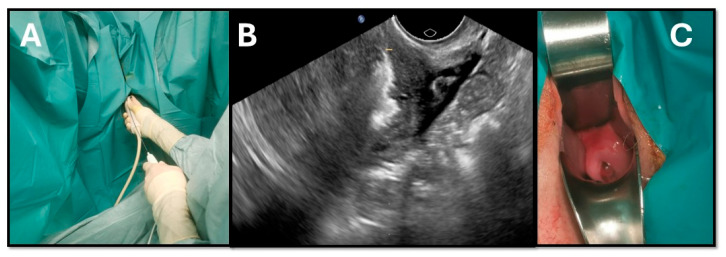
(A) Transducer with radiofrequency electrode. (B) Ultrasound appearance of ablation within the fibroid. (C) Transvaginal puncture sites after TRFA. Image created by the author.
